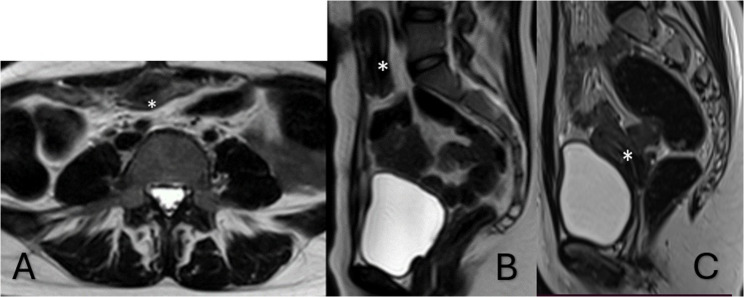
Axial (A) and Sagittal (B) T2W MRI after uterine transposition. Sagittal T2W MRI (C) then after repositioning of the uterus back into the pelvis. Uterine position is marked by asterisks
